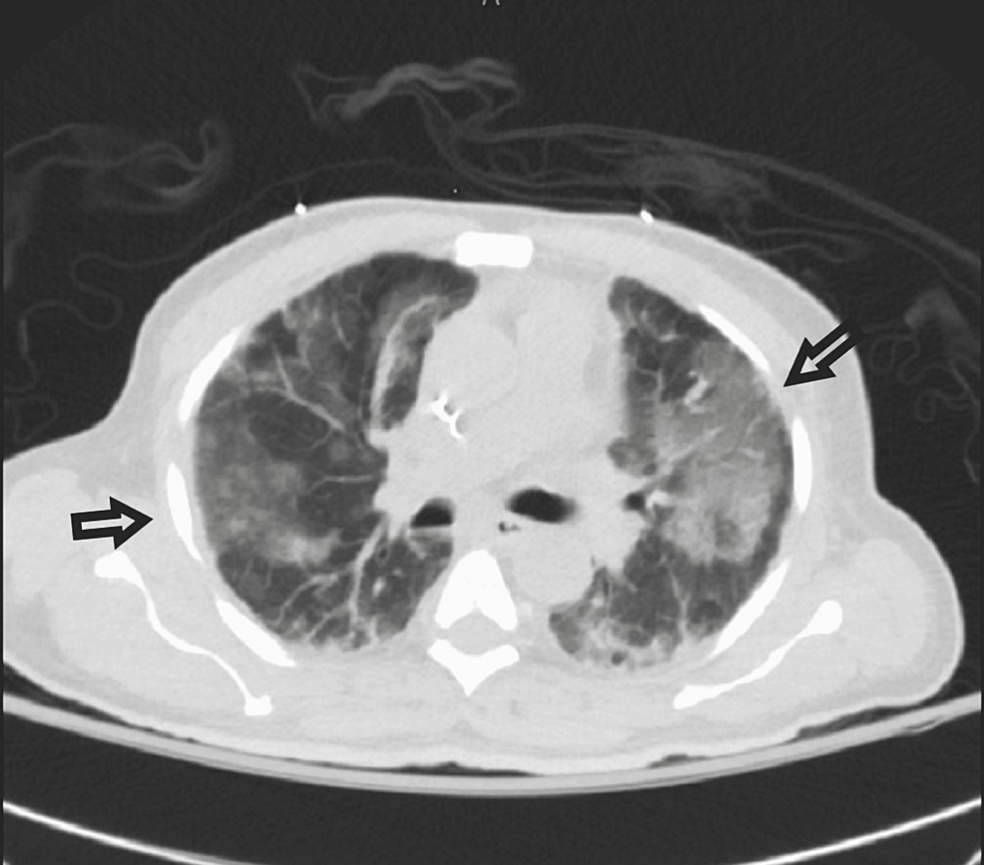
CT chest showing bilateral multiple pulmonary opacities (arrows).
